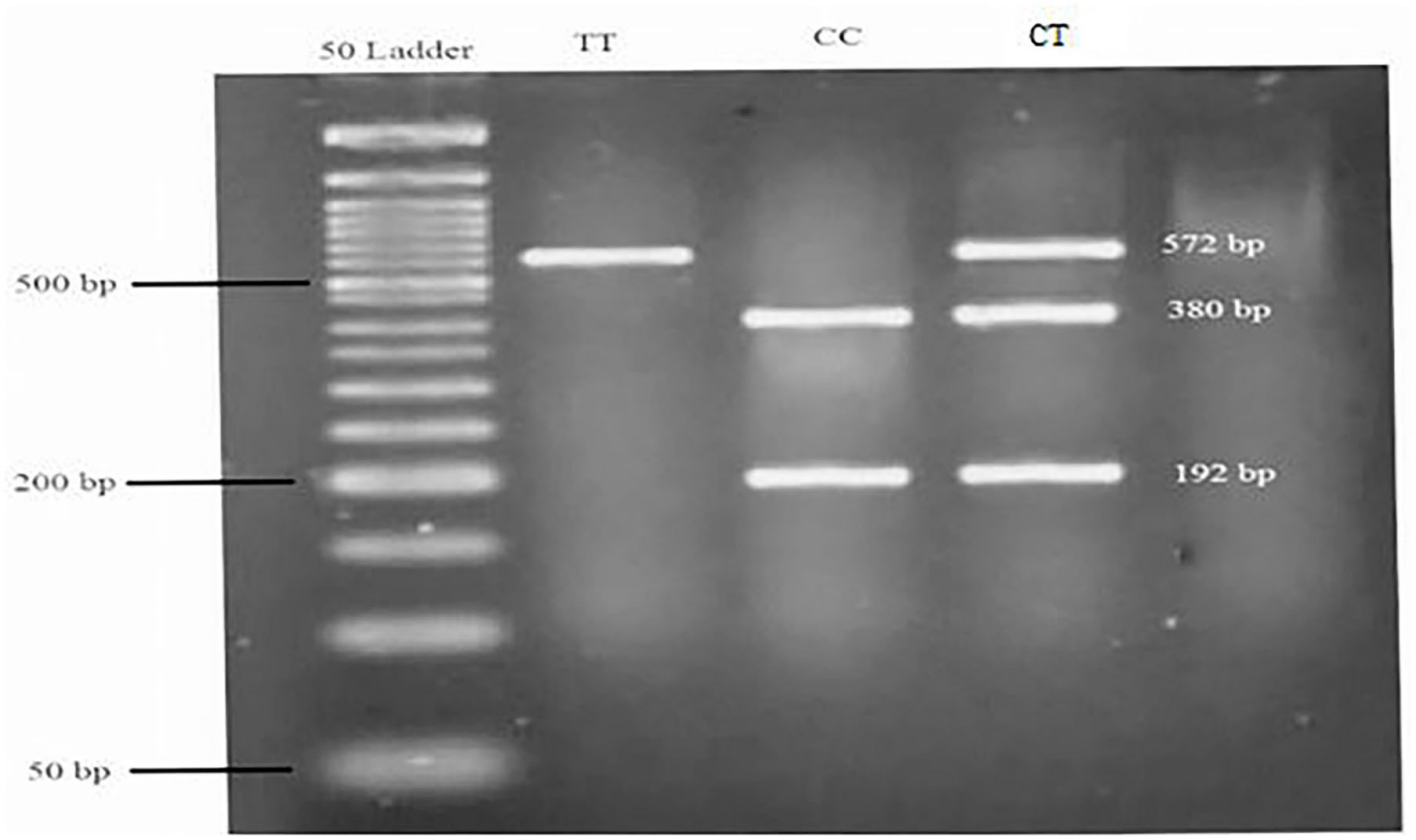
Genotyping of the FADS2 rs174583 variant by TauI PCR-RFLP analysis. A 50-bp ladder was used to determine the length of the digested products. TT = homozygous mutated (572 bp), CC = homozygous wild-type (380 and 192 bp), CT = heterozygous mutated (572, 380, and 192 bp).
